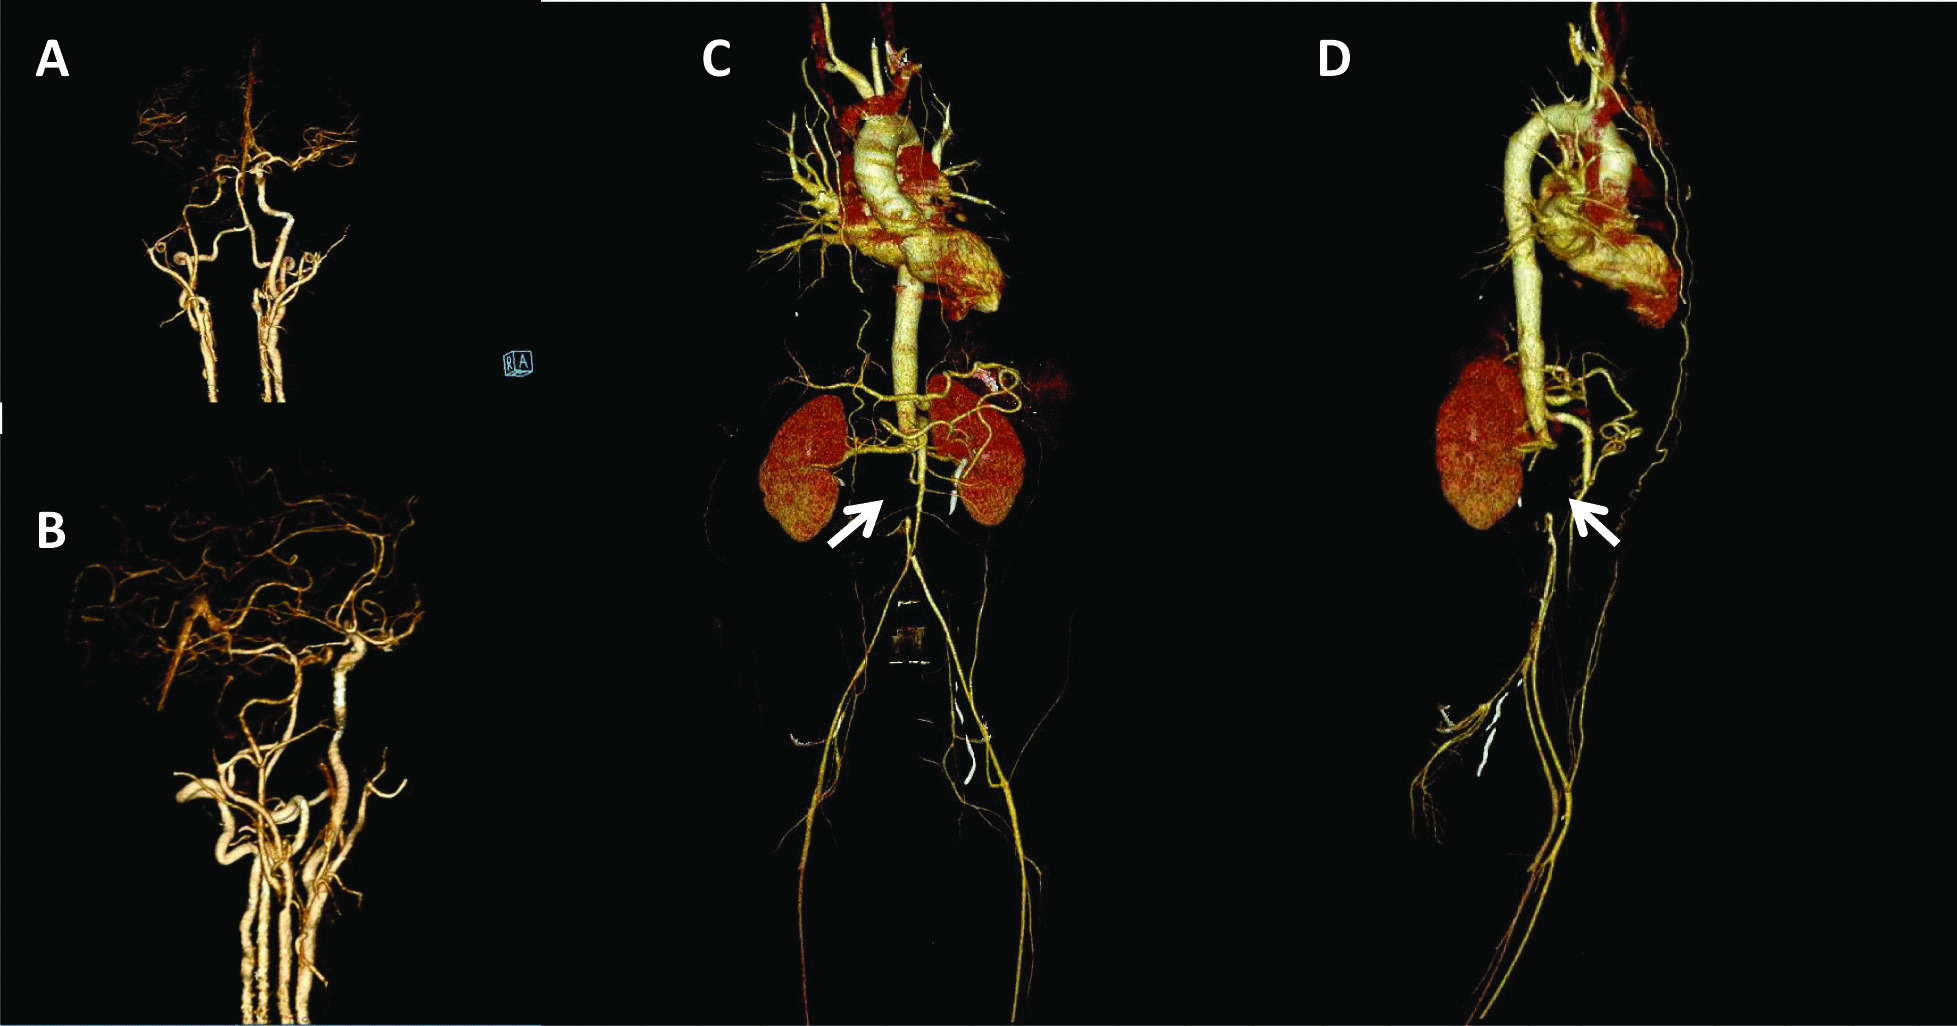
Pre-intervention computed tomography (CT) angiography of the patient’s stenosis of cerebral artery (A), carotid artery (B); CT angiography of abdominal and renal artery (C, D) complete occlusion of the abdominal aorta at the level of 10 mm above aortic bifurcation (Arrows)
